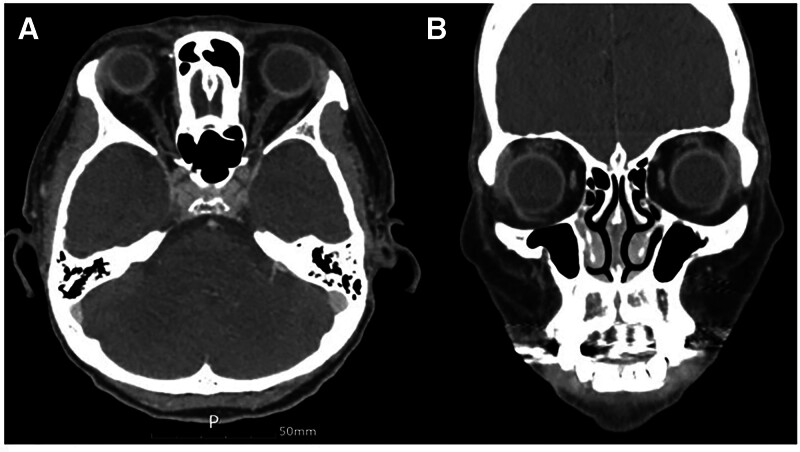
Postchemotherapy CT of the orbit. The axial (A) and coronal views (B) show a decrease in the size of the diffusely enlarged lacrimal glands. CT = computed tomography.
